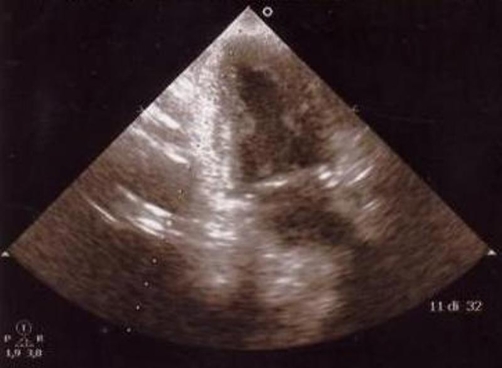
Transthoracic echocardiography (TTE) apical two chamber view.
